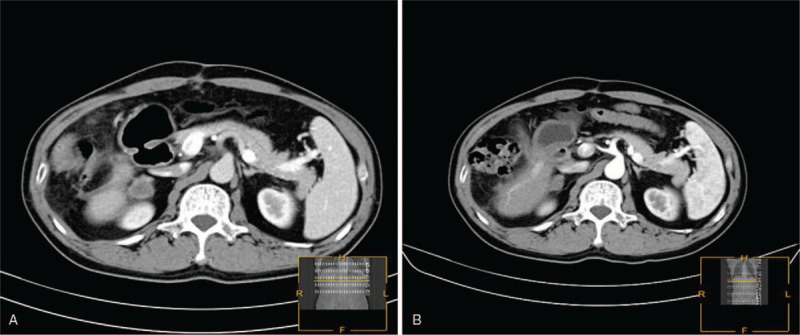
Images of the right adrenal metastasis 2 months after radical partial excision of the right liver lobe. (A) Enhanced CT scan shows a tumor in the right adrenal gland; (B) 3 months after radiofrequency ablation, the tumor had been completely ablated and devascularized, with the disappearance of tumor enhancement.
